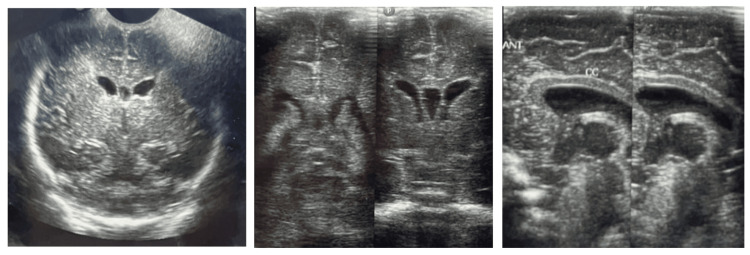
Ultrasonography of the brain done during the neonatal period showed no evidence of intraventricular hemorrhage.
